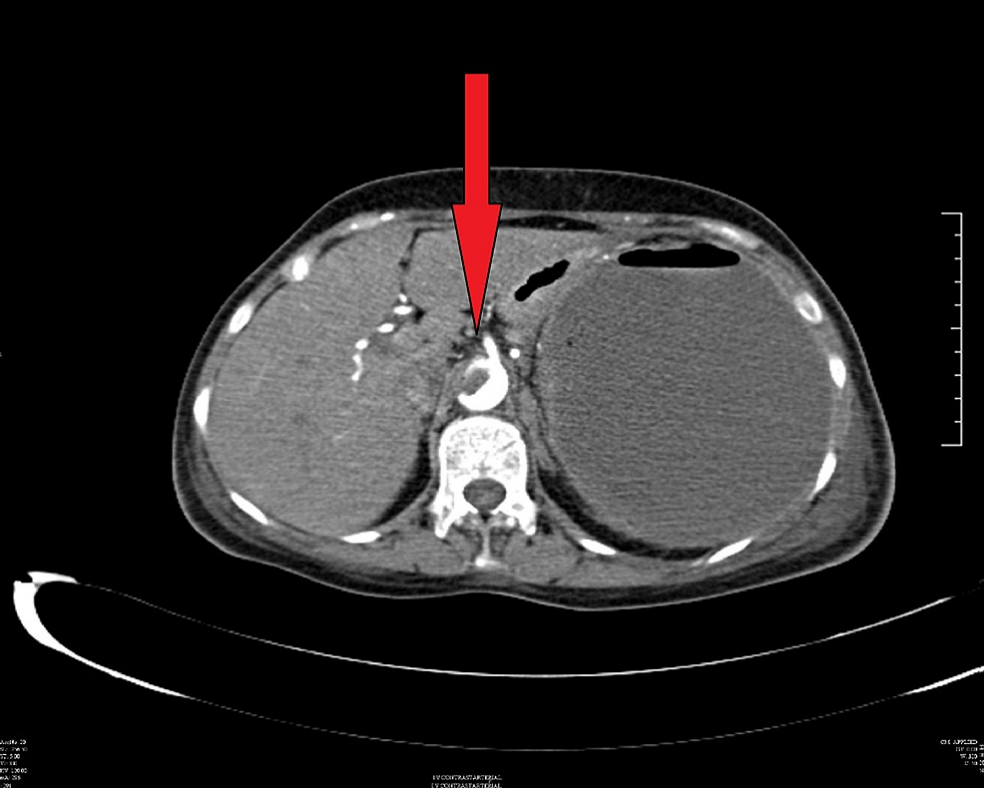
CT abdomen showing a filling defect in the abdominal aorta which is suggestive of a thrombus.
